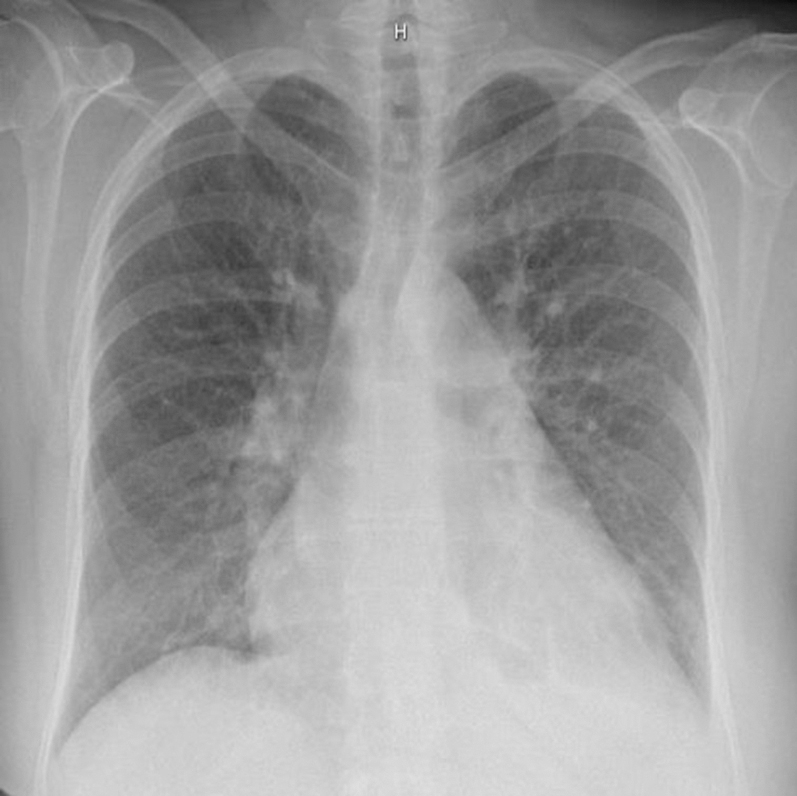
Initial Chest X-Ray The chest x-ray demonstrated cardiomegaly with mild pulmonary vascular redistribution.
